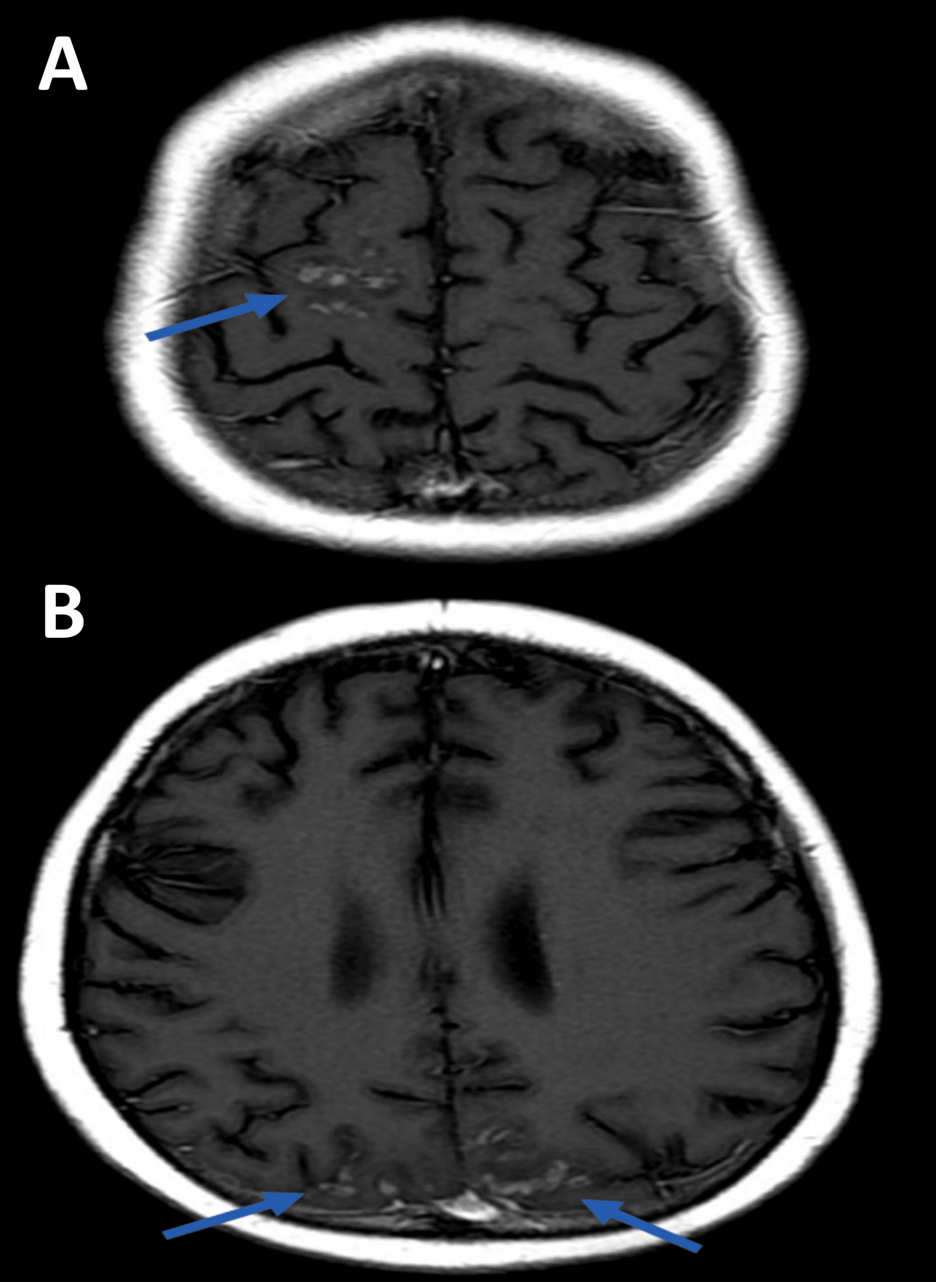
T1 post-contrast brain MRI images T1 post-contrast brain MRI images from the same child as Figure 4 demonstrate patchy enhancement in the right frontal and biparietal lesions (arrow) (A), but none in the left frontal lobe lesion (arrows) (B).
